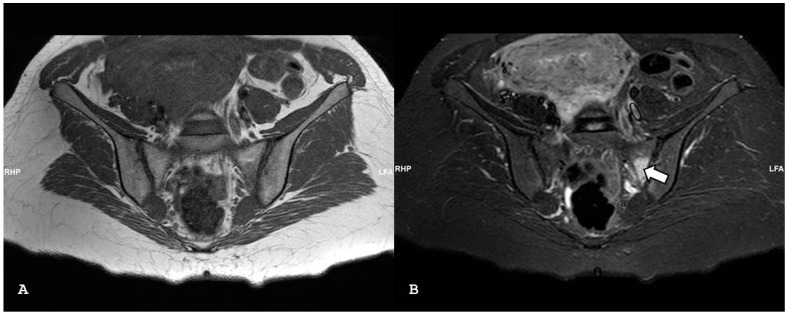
Oblique coronal T1-weighted (A) and oblique coronal short tau inversion recovery (B) magnetic resonance imaging sequences show focal patchy edema (arrow) at the mid-sacral area close to the left sacroiliac joint, in a woman who has recently given birth. No fracture line is evident.
